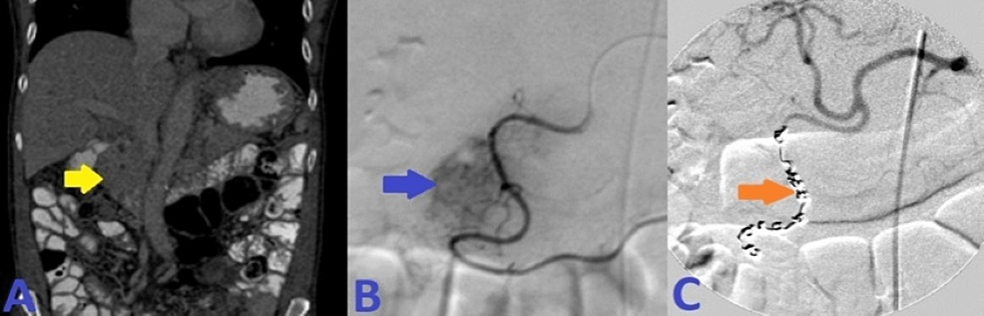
A: CT coronal image demonstrated duodenal fungating mass. B: Super-selective GDA angiograms using microcatheter System demonstrated many pseudoaneurysmal arteriolar tumoral feeders from the GDA branches. C: Post-embolization vascular catheterization for celiac angiogram demonstrated no evidence of active bleeding signs, and complete resolution of the above findings after coils' deployment in “sandwiching” appropriate positions, beyond the takeoff of main GDA, with adequate hepatic arterial inflow at the end of the embolization. CT: computed tomography; GDA: gastroduodenal artery.
